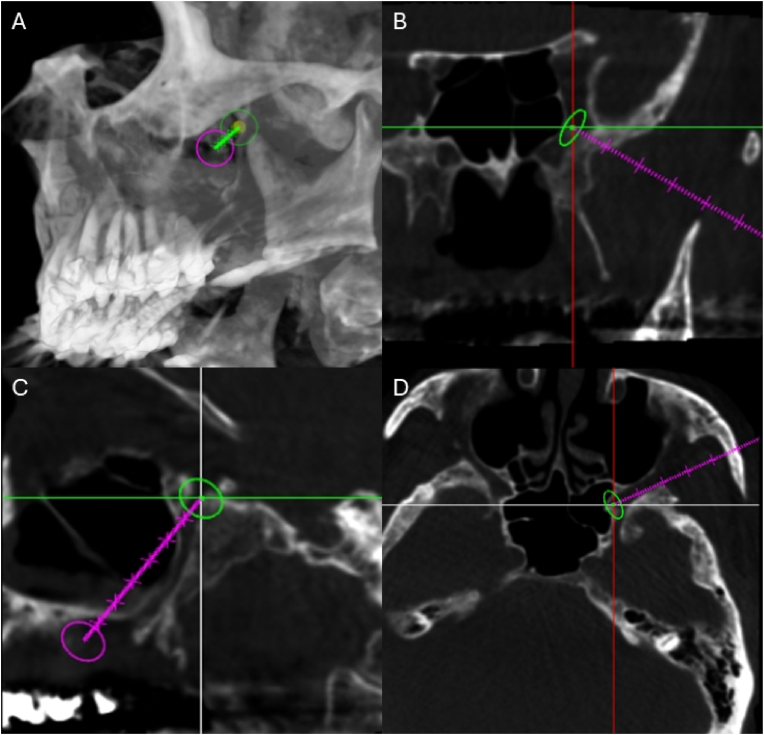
A) 3-D reconstruction of cone beam CT image used to determine safe trajectory for cryoablation probe. Green line: probe trajectory, green circle: probe tip, and red circle: probe tail. B) Coronal CT image demonstrating probe trajectory inferior to zygomatic arch with probe tip overlying pterygopalatine fossa. C) Sagittal CT image demonstrating probe tip overlying pterygopalatine fossa. D) Horizontal CT image demonstrating probe trajectory through pterygomaxillary fissure with probe tip overlying pterygopalatine fossa. B-D) Purple line: probe trajectory, green circle: probe tip. (For interpretation of the references to colour in this figure legend, the reader is referred to the Web version of this article.)
